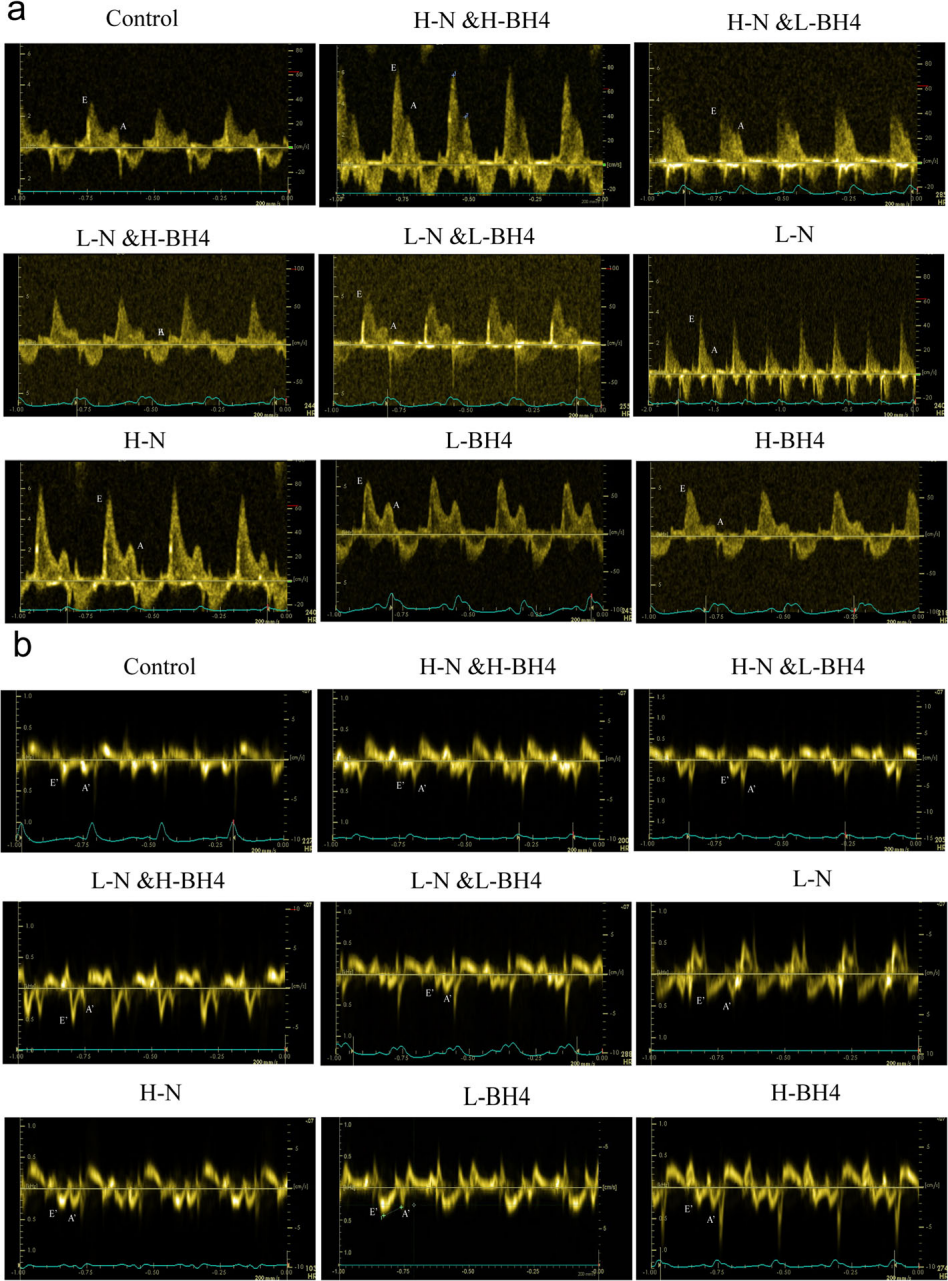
Comparison of cardiac function by colour ultrasound. a E and A peaks in each group. b Comparison of E ‘/A’ among each treatment group and the control group
